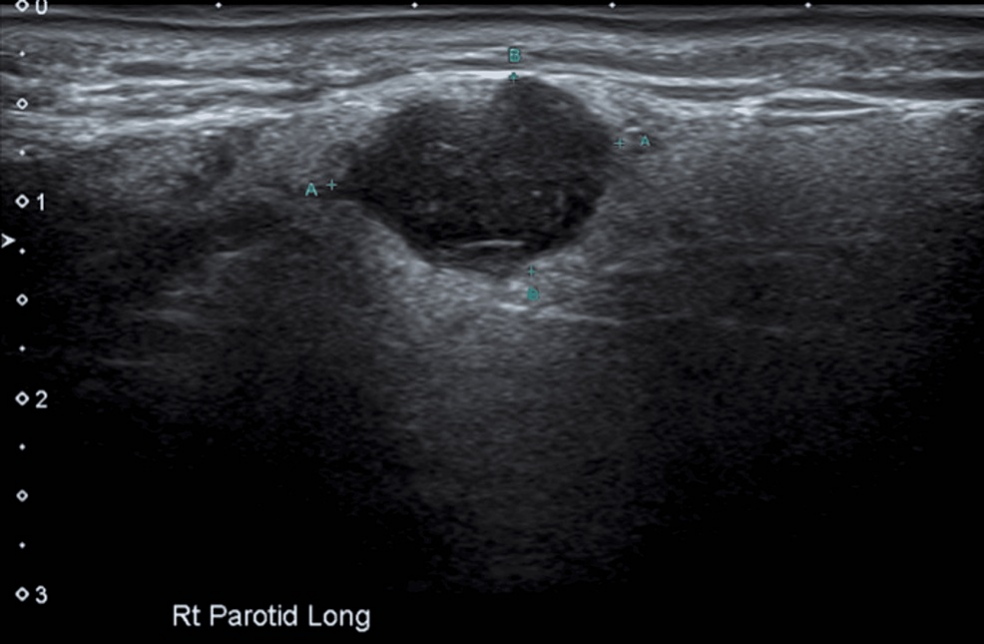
Ultrasound of right parotid gland showing a 14 mm × 11 mm × 12 mm cystic lesion
